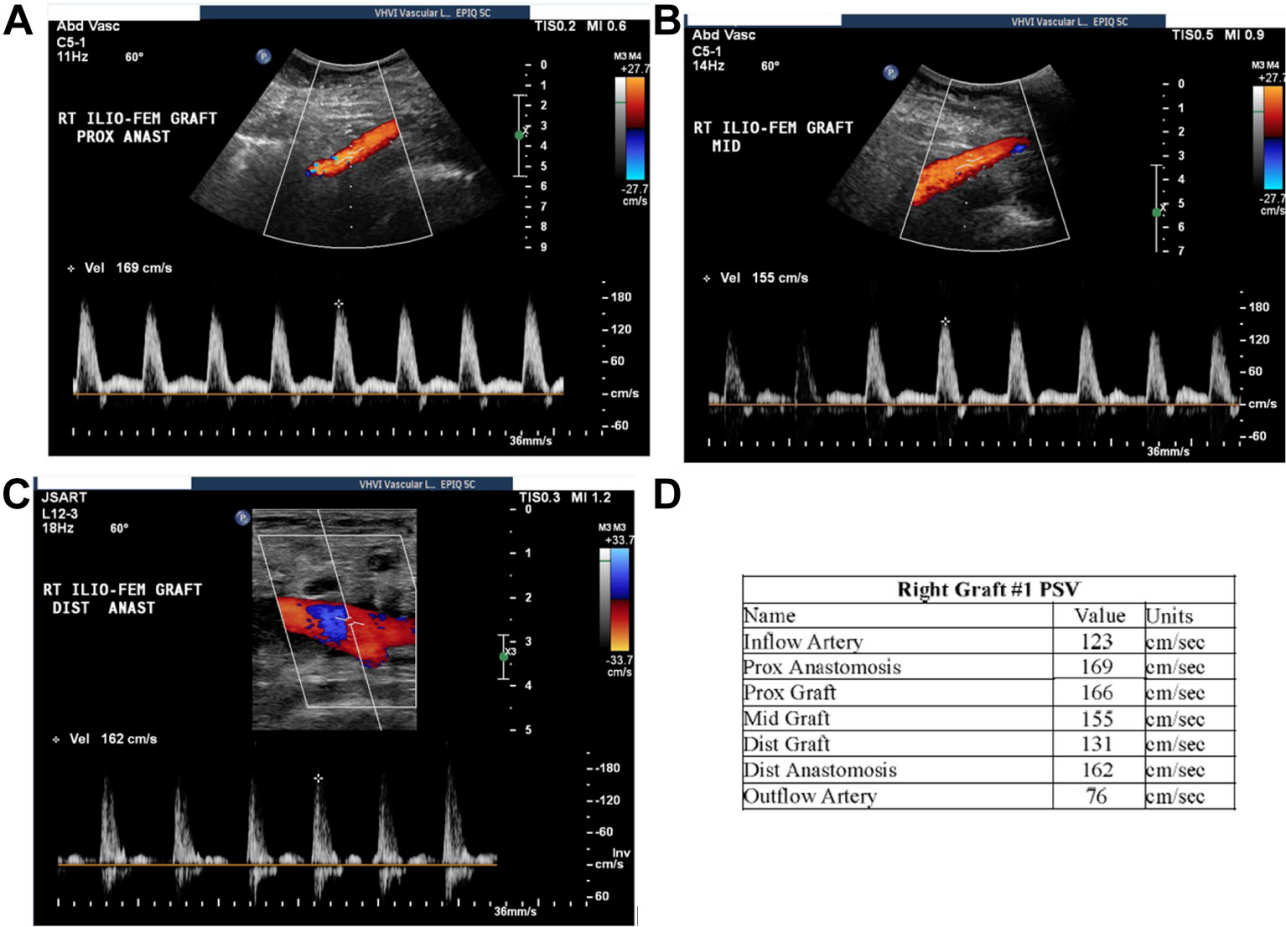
Selected duplex ultrasound images at 1 month after human acellular vessel (HAV) implantation for the proximal anastomosis (A), midgraft (B), and distal anastomosis (C). D, Peak systolic velocity (*PSV*) values for the right graft. *Anast,* Anastomosis; *Dist,* distal; *Ilio-Fem,* iliofemoral; *Prox,* proximal; *Rt,* right.
